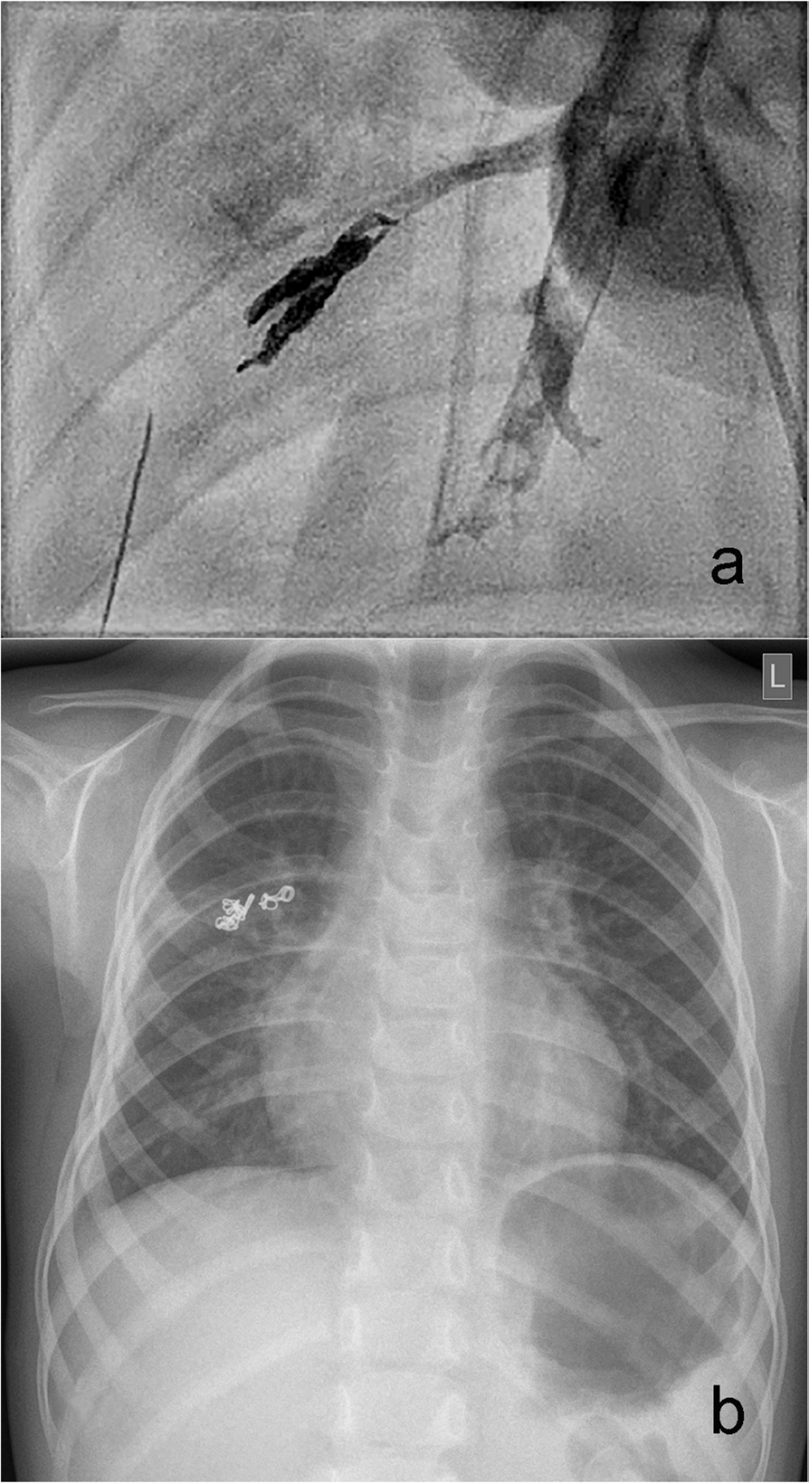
a Coiling Bronchography after coiling PAL in bronchus 3r. b Chest Xray control 3 months after endobronchial intervention
